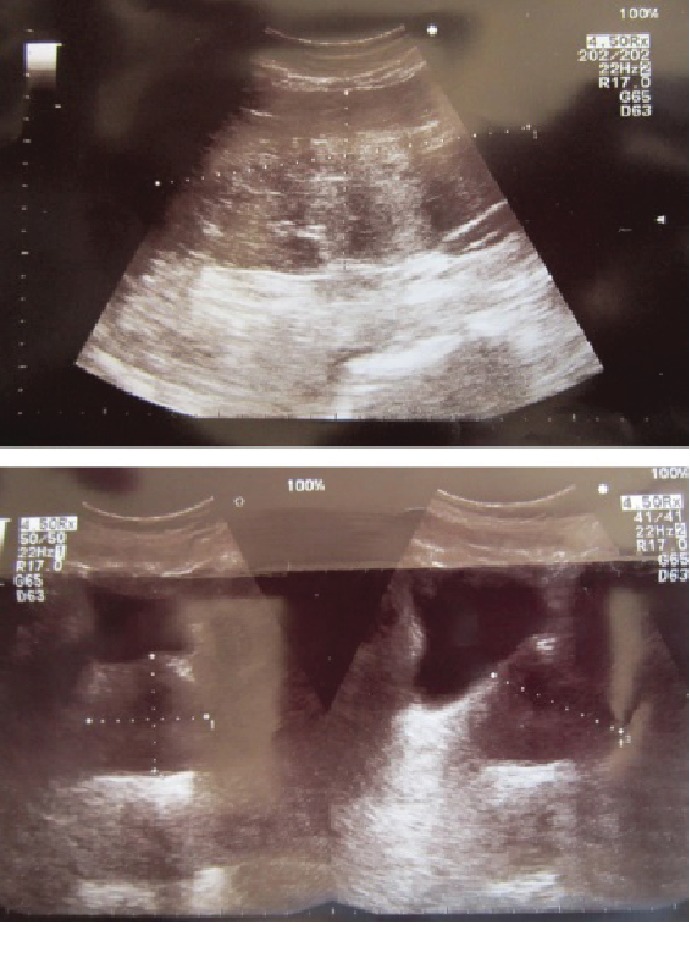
Ultrasound of abdomen showing big hyperechoic intra-abdominal mass.
